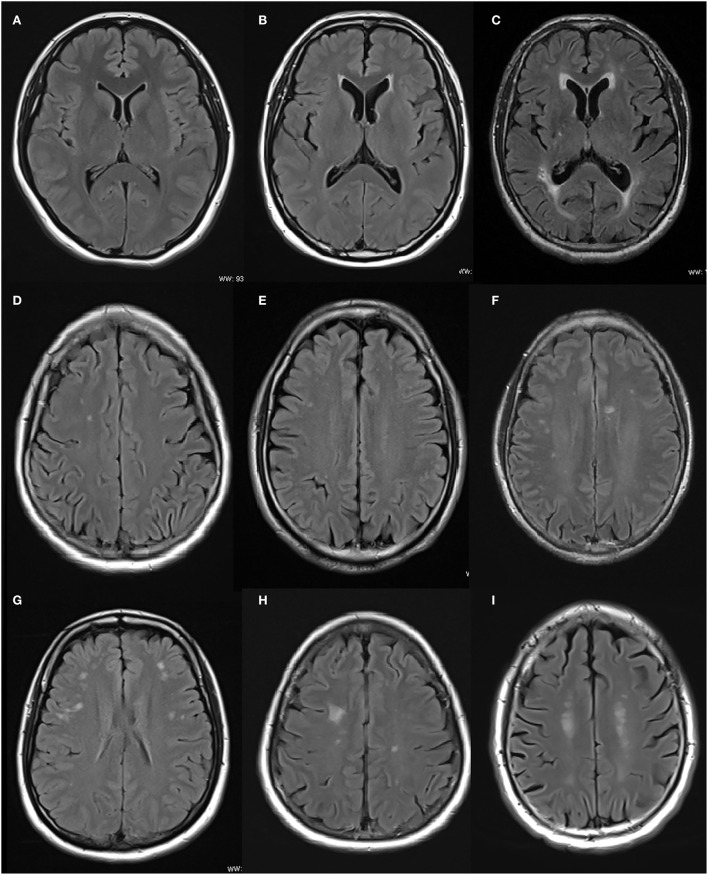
Examples of axial FLAIR images of WMHs' score in frontal horn and frontal lobe. (A) Scored 0 point since there were no PWMHs in the frontal horn and occipital horn. (B) Scored 1 point since the PWMHs in the frontal horn and occipital horn were <5 mm. (C) Scored 2 points since the PWMHs in the frontal horn and occipital horn were between 5 and 10 mm. (D) Scored 1 point since the number of lesions in the frontal lobar was <5 and the largest one was smaller than 3 mm. (E) Scored 2 points since the number of lesions in the frontal lobar was more than 5 and the largest one was smaller than 3 mm. (F) Scored 3 points since the number of lesions in the frontal lobar was <5 and measured between 4 and 10 mm. (G) Scored 4 points since the number of lesions in the frontal lobar was more than 6 and measured between 4 and 10 mm. (H) Scored 5 points since there was a lesion measured larger than 11 mm. (I) Scored 6 points since there were confused lesions in the frontal lobar.
